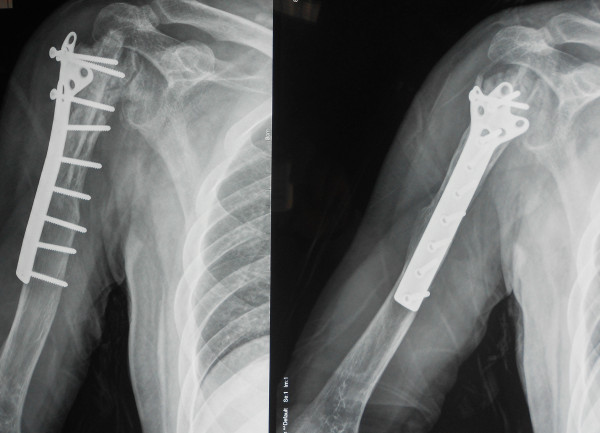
Post operative X-ray showing adequate union of the junction.
